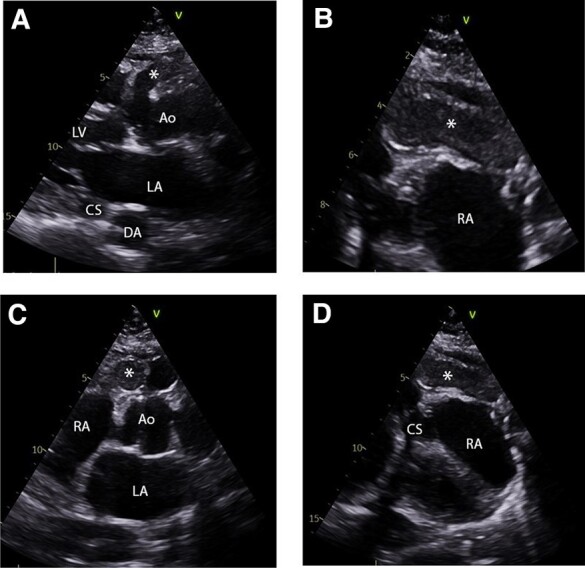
Post-operative transthoracic echocardiogram (TTE) images demonstrating thrombosed segments of the RCA (marked as asterisk) following successful closure of the fistula and concurrent saphenous venous graft (SVG) to posterior descending artery (PDA) of the RCA. RA, right atrium; LA, left atrium; Ao, ascending aorta; LV, left ventricle; DA, descending aorta; CS, coronary sinus.
